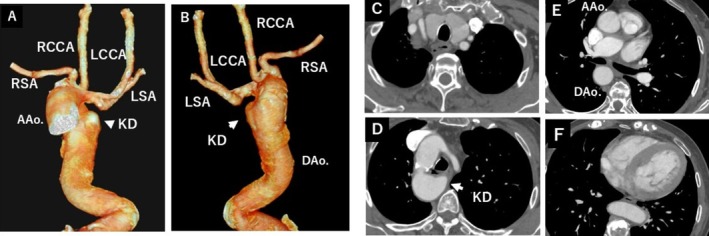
Computed tomography (CT) in Case 2. (A, B) Three‐dimensional volume‐rendered CT images from anterior and posterior views, respectively, demonstrating a right aortic arch with a large Kommerell's diverticulum (KD, indicated by arrowhead/arrow). (C–F) Axial CT images demonstrating the anatomy of the aortic arch and the KD (arrowhead in D).
